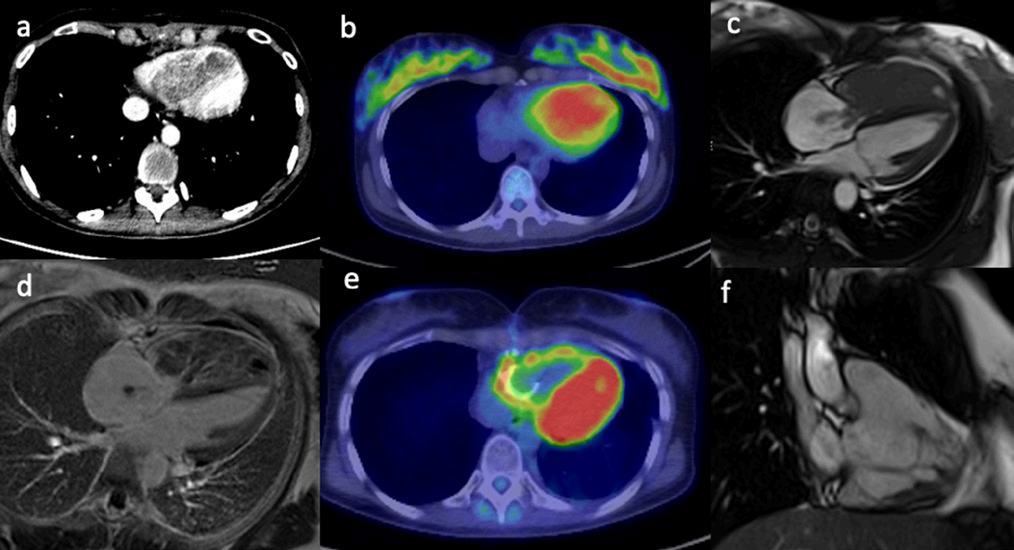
(a) Contrast-enhanced CT scan shows an ill-defined low
